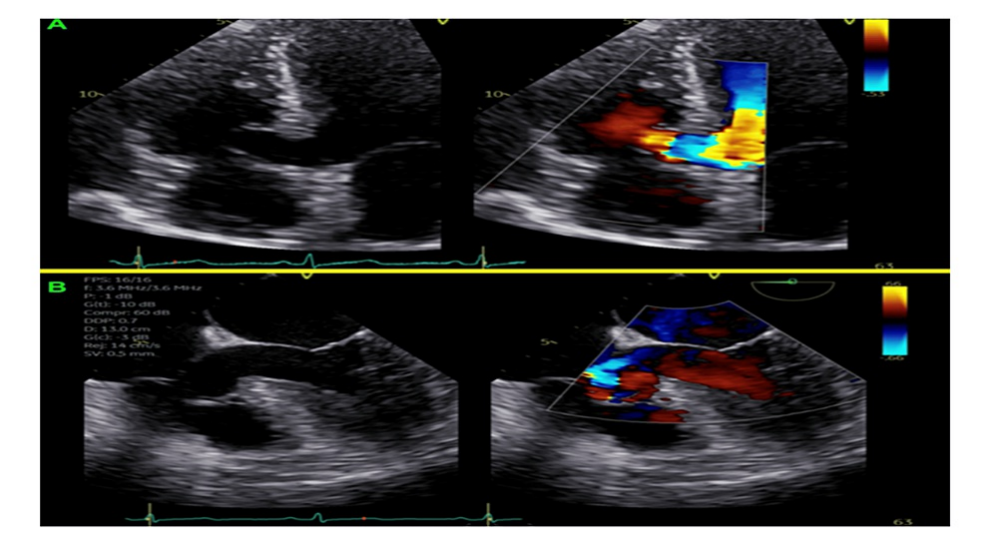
(A)Transthoracic echocardiogram revealing a turbulent flow within basal interventricular septal aneurysm without intracardiac shunting. (B) Trans-esophageal echocardiogram demonstrating a basal IVS aneurysm measuring 12.2 mm × 16 mm without intracardiac shunting or thrombi.
